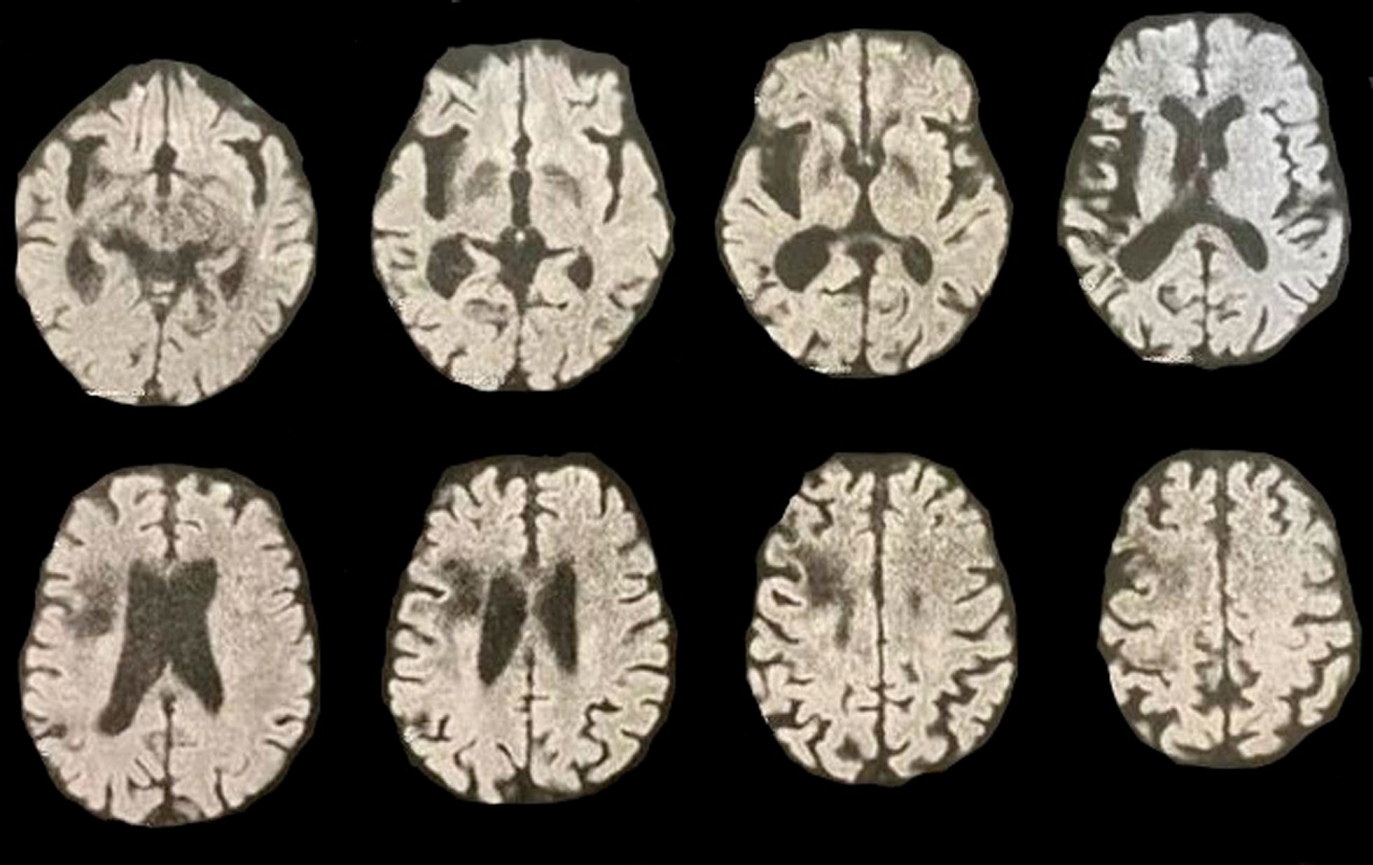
Baseline magnetic resonance imaging diffusion film of the presented case showing dilated right insular ribbon and ipsilateral posterior horn of lateral ventricle donating the patient’s old stroke
